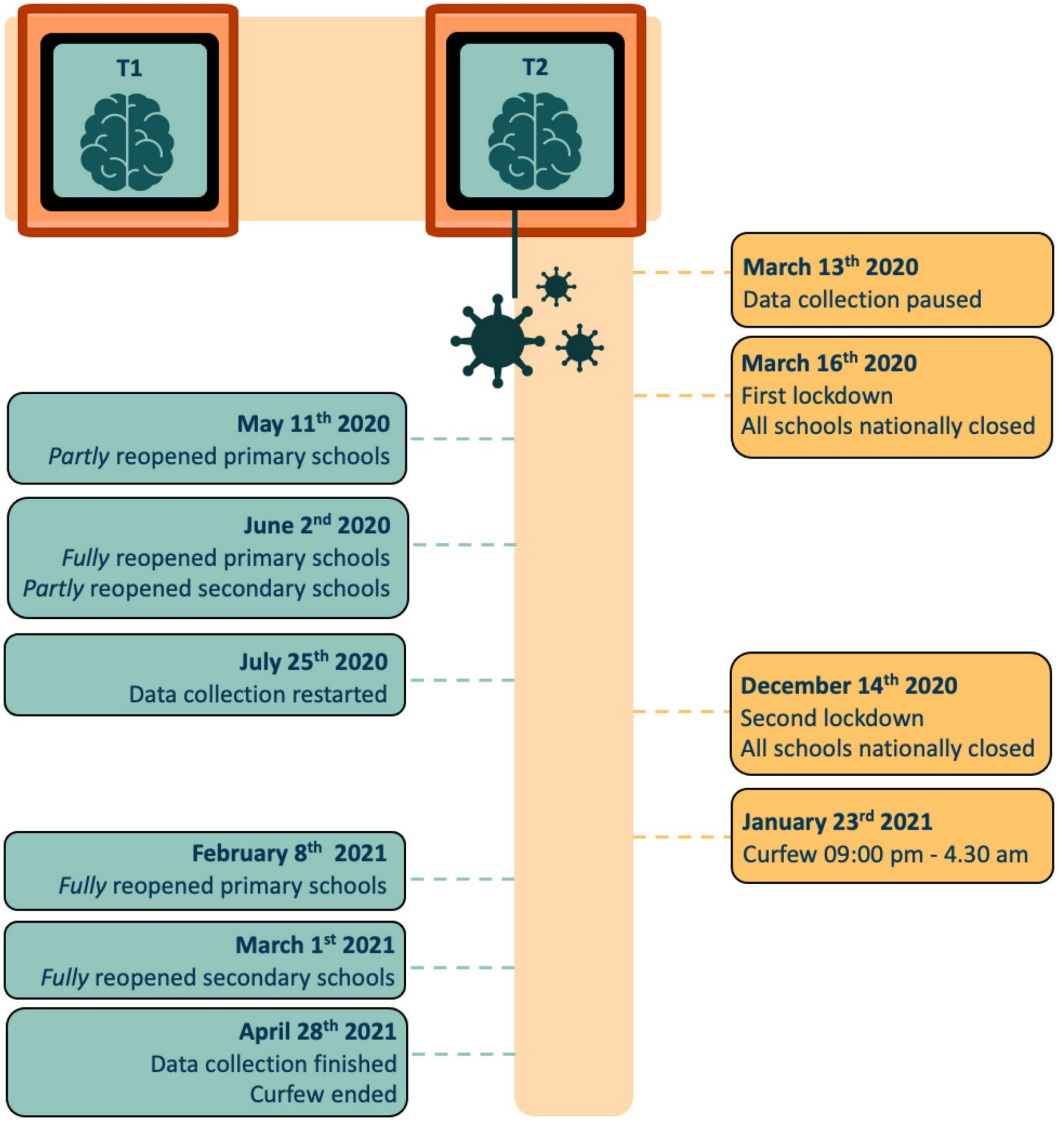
Timeline of COVID-19 pandemic measures in the Netherlands during data collection of MRI time point 2. Note that the strict restrictions of the COVID-19 pandemic are depicted in orange on the right and the more lenient restrictions are depicted in turquoise on the left. T1 = time point 1; T2 = Time point 2.
